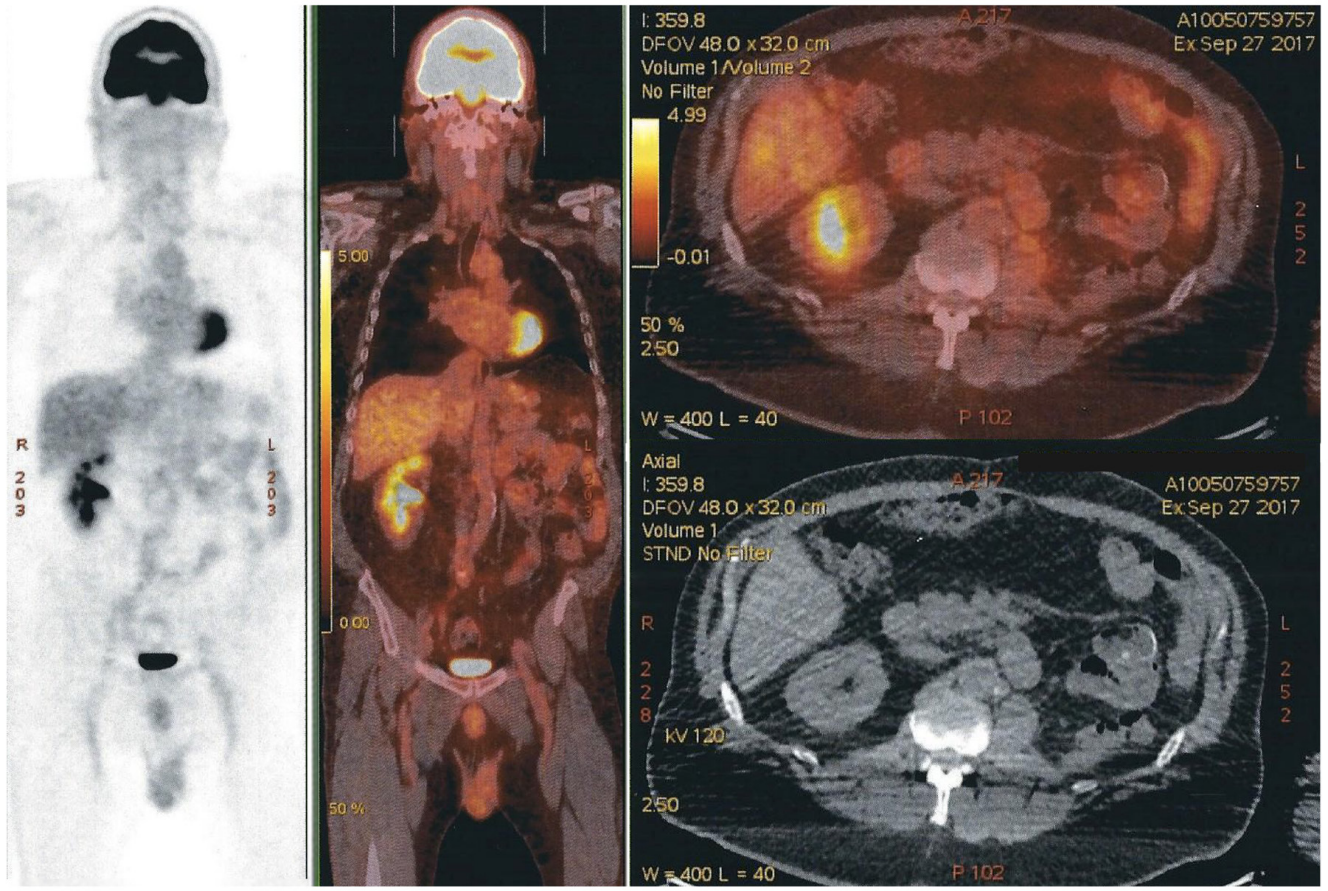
PET-CT scan with no evidence of recurrence of oncologic disease. Free left renal-bed, absence of distal lymph nodes, and nonexistence of any new suspect lesion. The modified area on the right side of the image is a shape added to cover the name of our patient.
